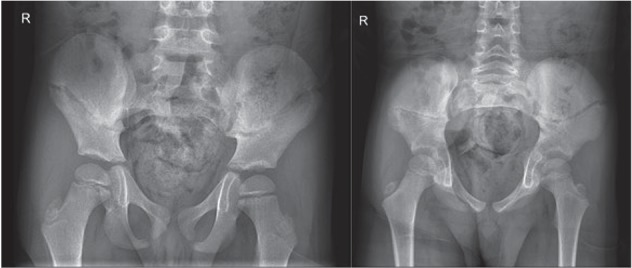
Radiography with formation of callus visible after six weeks.
